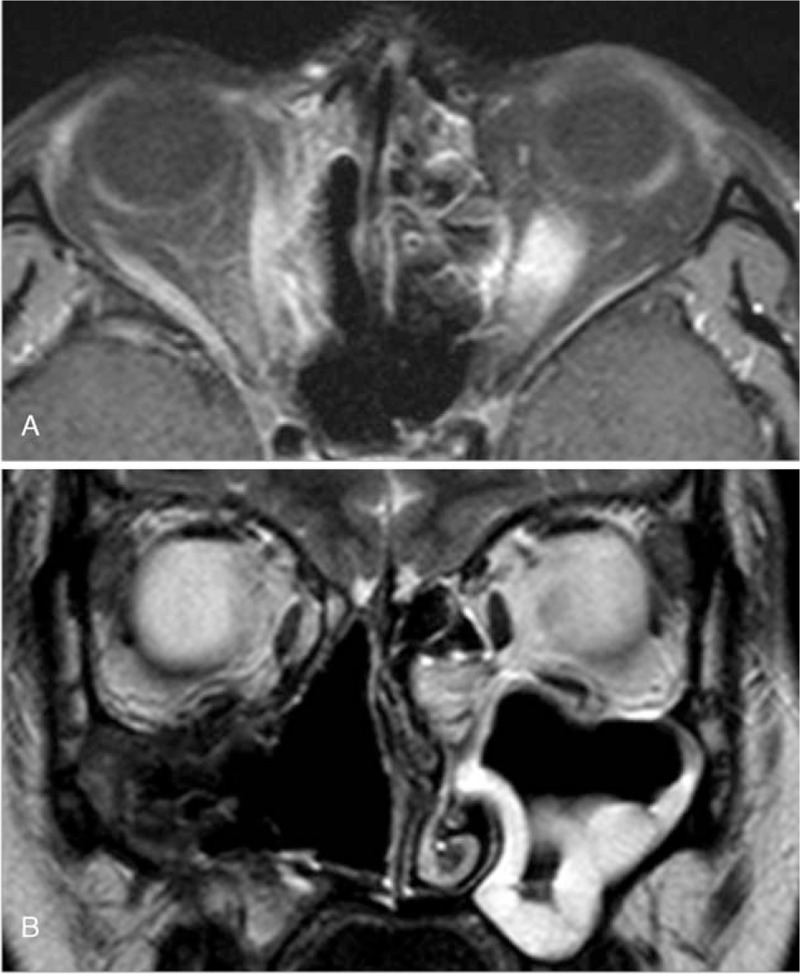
Axial (A) and coronal (B) magnetic resonance imaging showing postoperative right maxillary mucosal wall thickening and left maxillary sinusitis 1 year after treatment.
